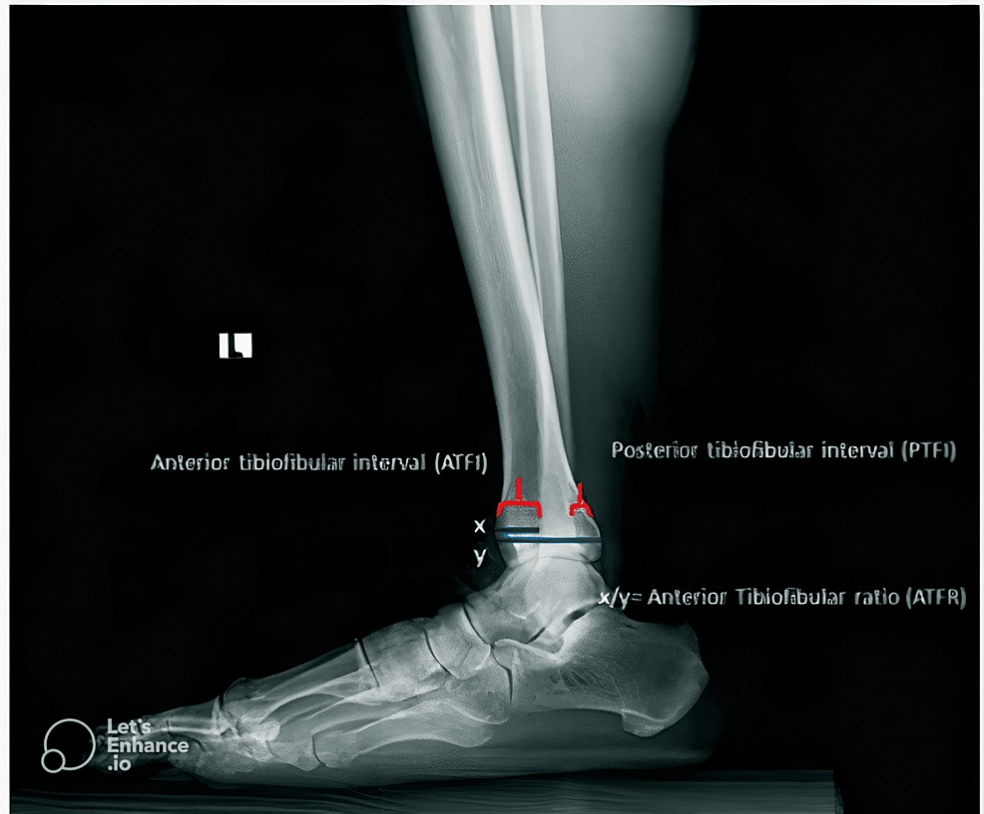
Lateral radiograph measurements
